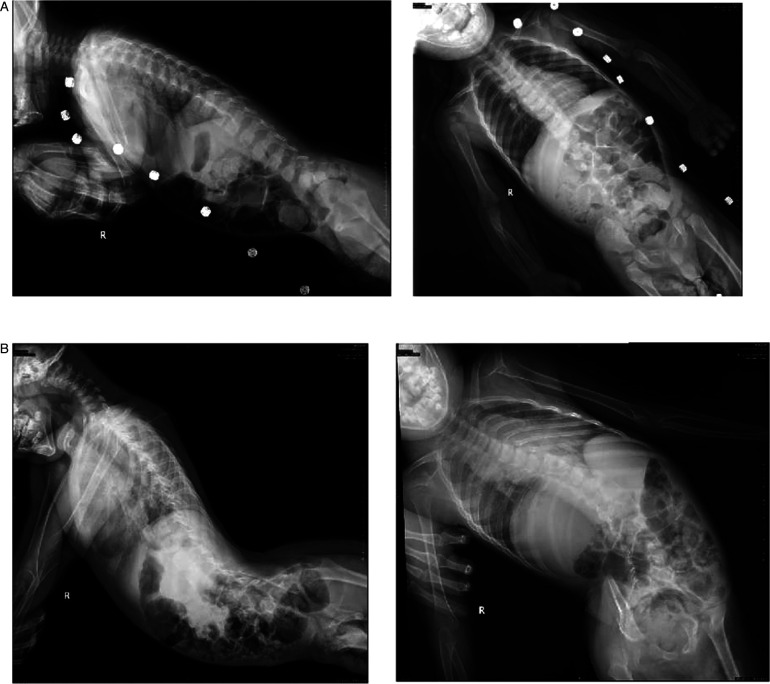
The spine x-rays. (A) The spine x-rays of the proband; (B) the spine x-rays of the elder brother. The spine x-rays showed that the spine of the proband was normal and the elder brother had severe scoliosis and cervical kyphosis.
